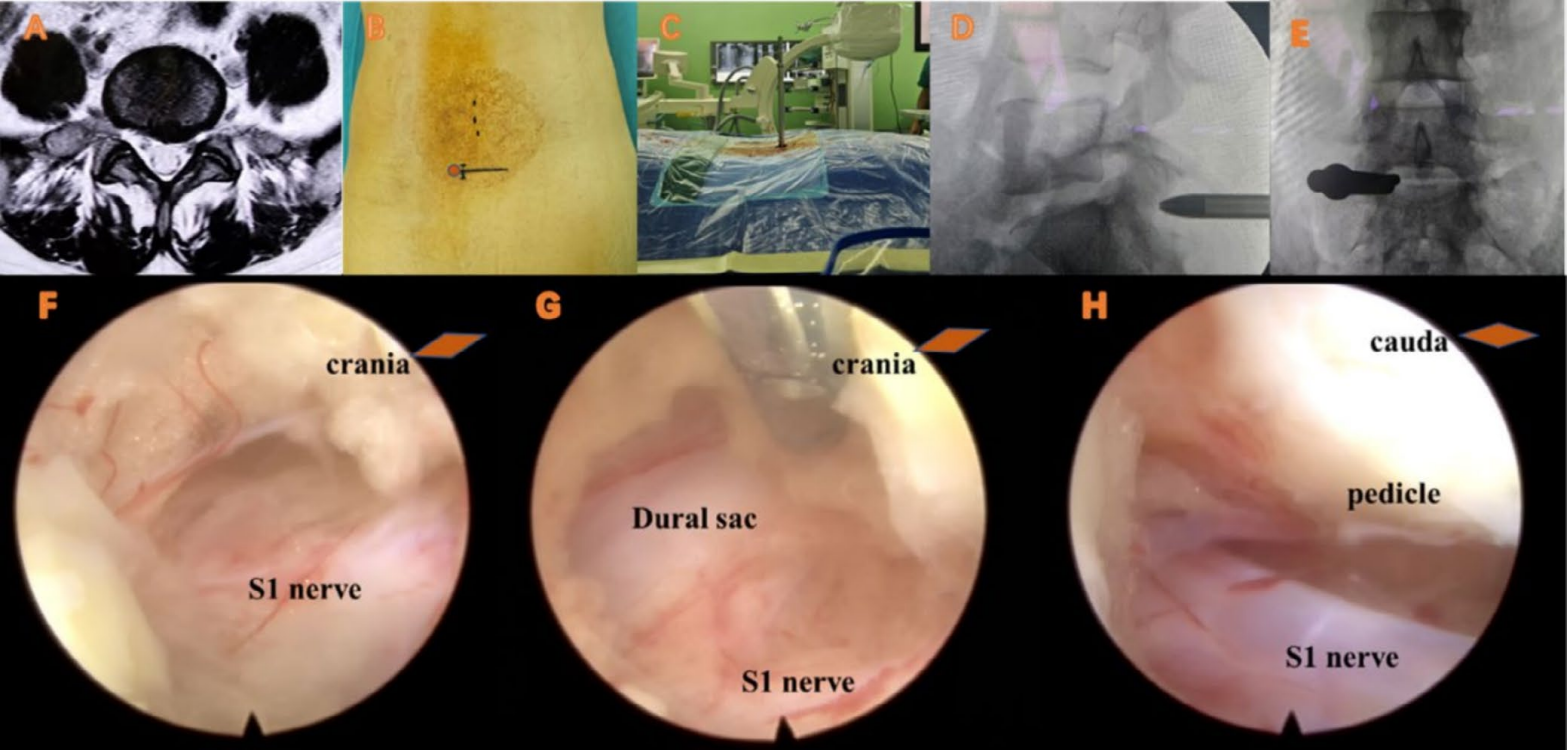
A young patient (age: 30–40 years old) presented with chronic radiating pain in the left lower extremity for 1 year, which had worsened for 1 month, and the operation time was 34 min. (A) Preoperative MRI suggested a left lumbar disc herniation at L5/S1. (B) The intersection of the superior endplate and the facet joint (orange dot) was used as the puncture target (facet joint degeneration was mild, and the incision was moved inward by 2 mm). (C) Insert the pencil tip vertically and establish the channel. (D, E) Fluoroscopy verification. (F) After removing a little ligamentum flavum, the vertically placed channel leads directly to the S1 nerve. (G) S1 nerve and dura sac were identified after cutting the ligamentum flavum. (H) S1 nerve and pedicle visible (flip lens).
